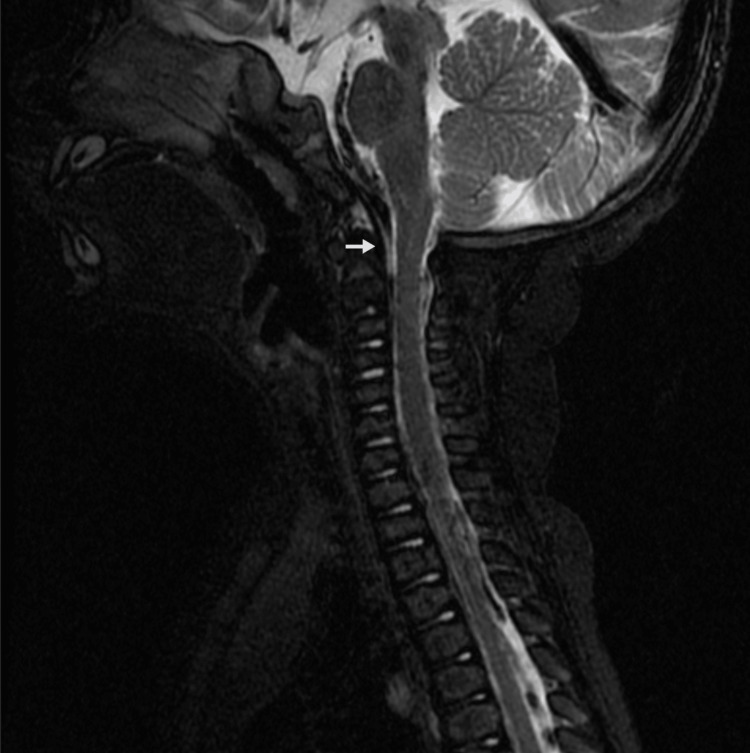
Cervical spine MRI (sagittal view) of the patient. The image shows a reduction in the caliber of the occipital foramen but without signs of medullary parenchymal molding (arrow).
